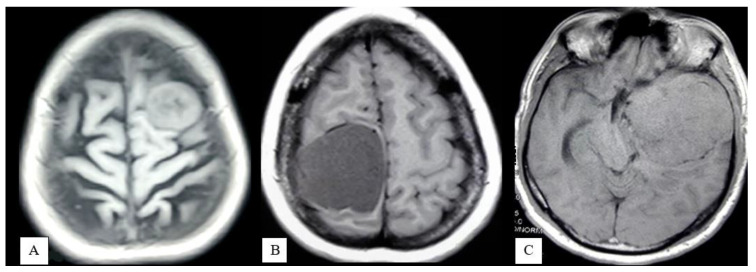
MRI of brain T1-WI axial section demonstrates small (A), medium (B), and large size meningioma (C).
